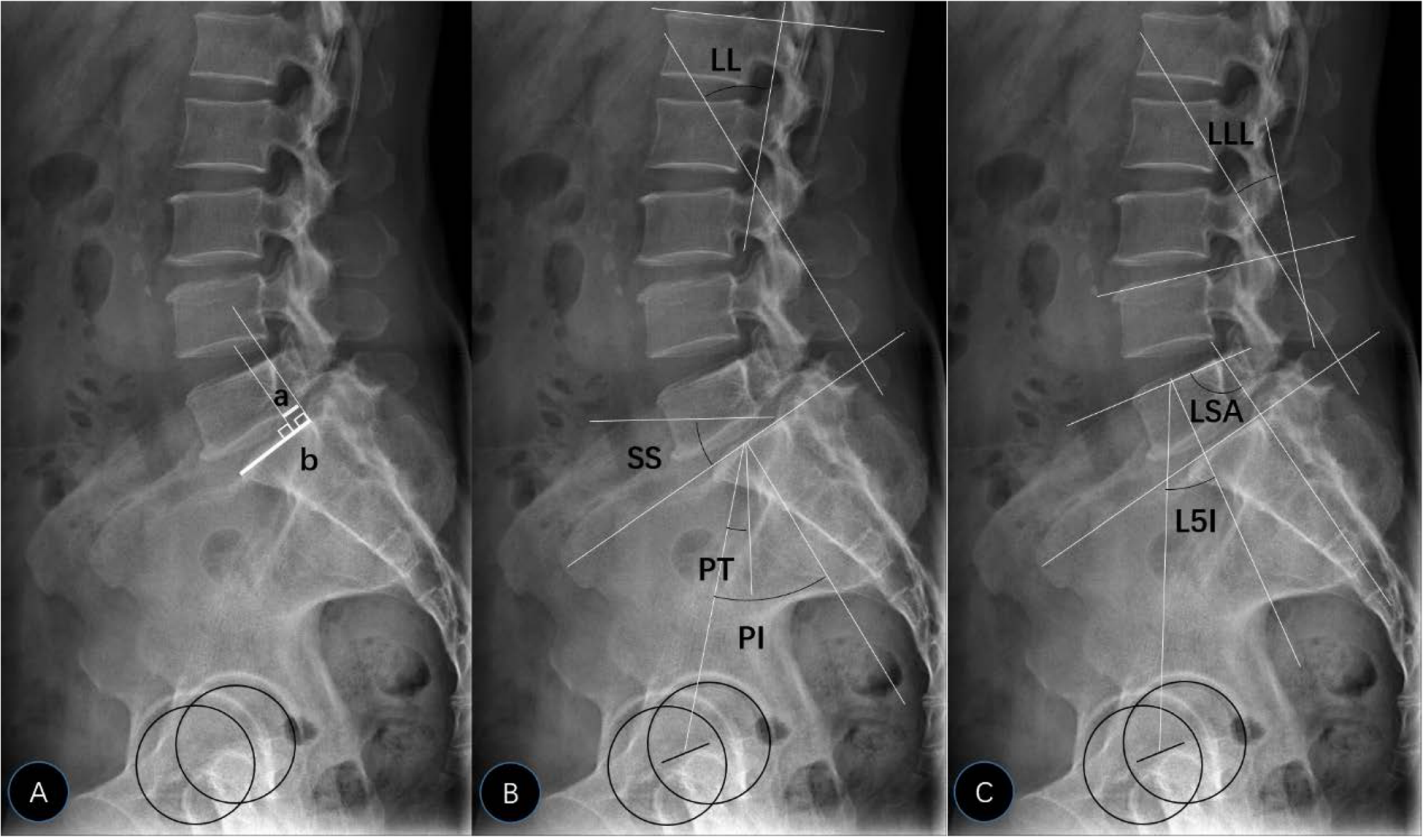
Measuring method of parameters of lateral X-ray film. a Measuring method of SR: SR = a/b*100%. (a: distance between the vertical line of superior endplate of the lower vertebra through posterior end of inferior endplate of upper vertebra and the vertical line of superior endplate of the lower vertebra through posterior end of superior endplate of lower vertebra. b: length of superior endplate of lower vertebra.) b Measuring method of PI, PT, SS, and LL. c Measuring method of LLL, LSA, and L5 I
